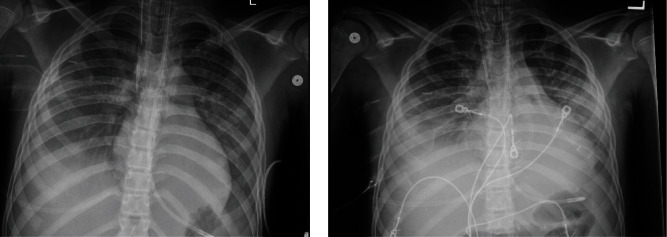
Chest radiograph one day prior (a) and at day of arrest (b).
